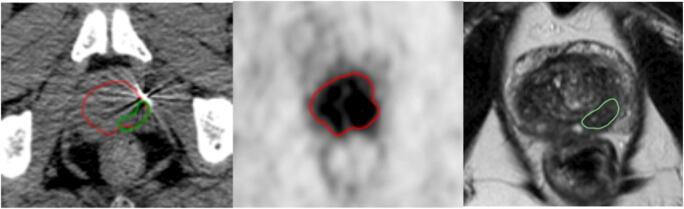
Example of differences in tumor volumes and laterality. (Left) axial CT slice with fused gross tumor volumes (GTV) delineated in PET (GTV-PET, red) and mpMRI (GTV MRI, green). (Middle) GTV delineated in PSMA-PET (scaling SUVmin−max 0–5). (Right) GTV delineated in mpMRI, image shows axial T2-weighted MR image. GTV-PET is larger than GTV delineated in MRI. Additionally, the GTV-PET extents to the right and left lobe, whereas the GTV-MRI is restricted to left lobe only
